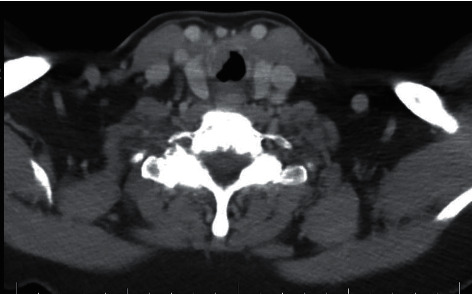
Axial CT neck with contrast demonstrating a right subglottic mass.
